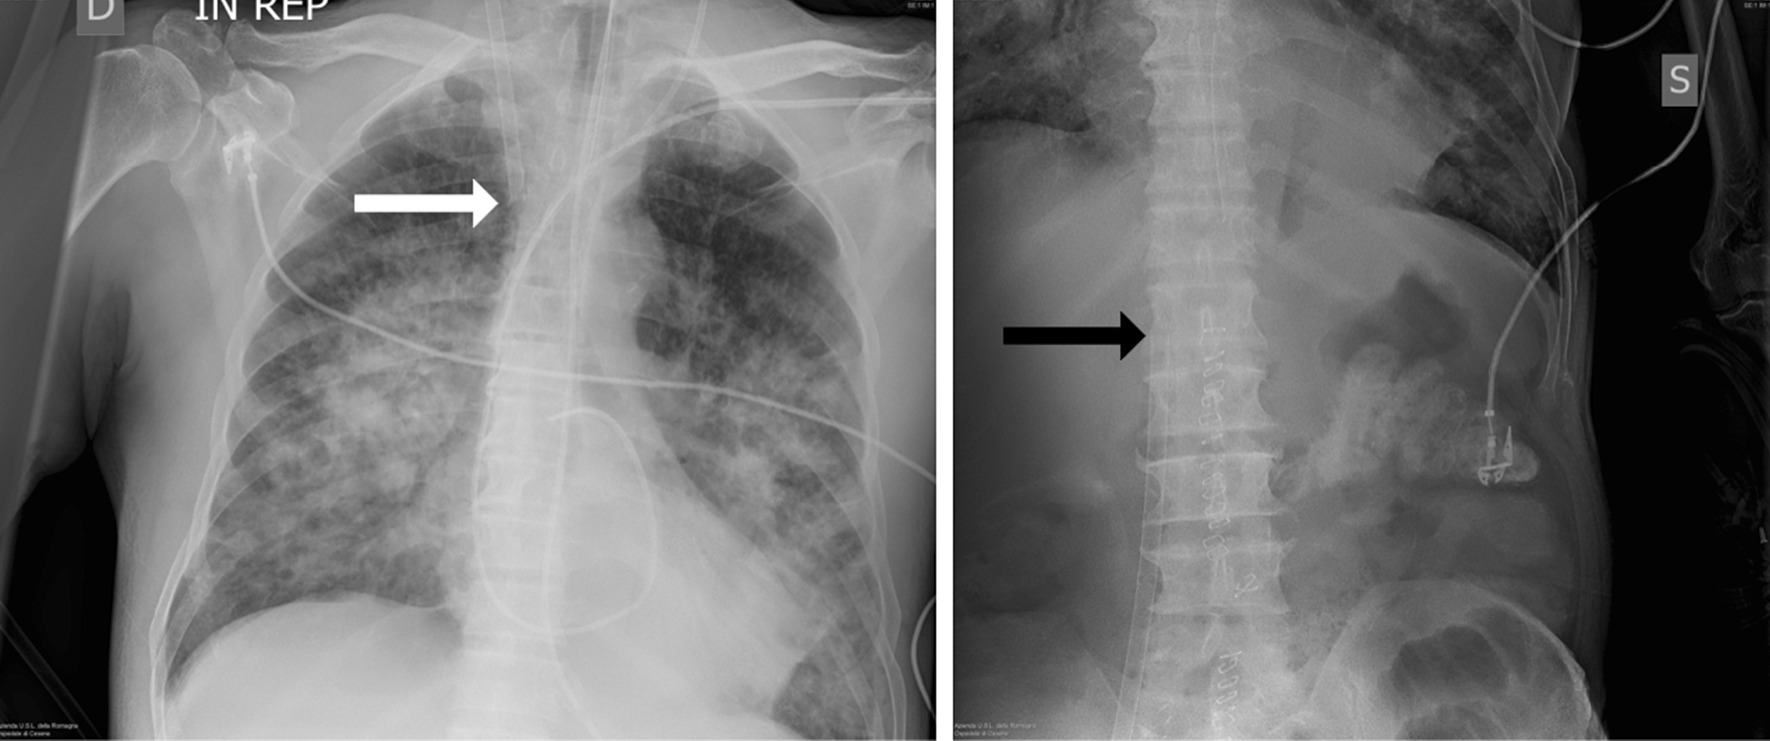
X-ray control for placement of cannulas for veno-venous extracorporeal membrane oxygenation. White arrow pointing the tip of the return cannula in superior vena cava while black arrow pointing the tip of the drainage cannula in inferior vena cava
